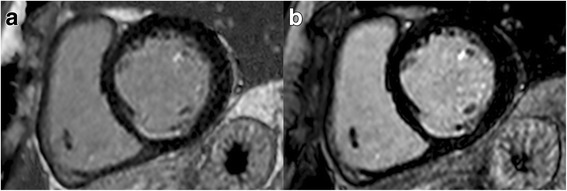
2D turbo-field-echo inversion recovery T1-weighted basal short-axis images showing a subendocardial scar in inferoseptal segment. Good contrast between subendocardial border and LV blood pool is obtained after intravenous injection of gadobutrol 0.1 mmol/kg with a time delay of 10 min (a) as well as after injection of a 0.2 mmol/kg dose with a time delay of 20 min (b)
